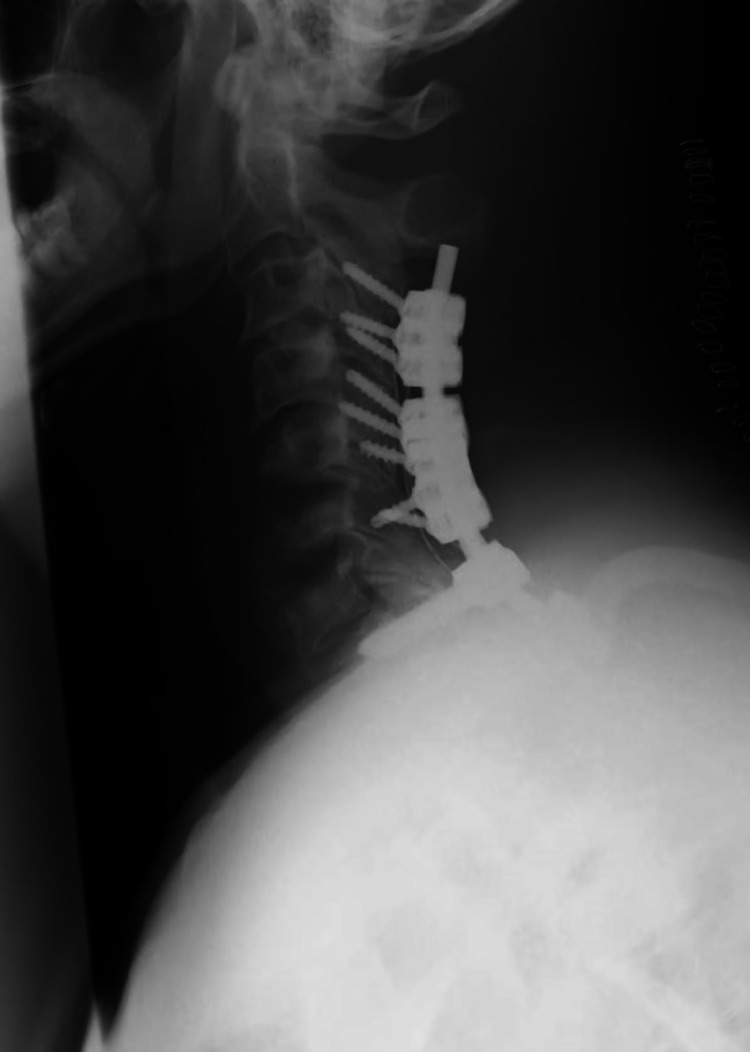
Cervical radiography after the second surgery Lateral view of the cervical radiograph after posterior fixation. Lateral mass screws were placed bilaterally at C3, C4, and C5 and on the left side at C6. A transarticular screw was placed on the right side at C6 due to the small lateral mass. Pedicle screws were placed bilaterally at Th1 and Th2. The pedicle screws at C7 were skipped because of pedicle fragility due to the spread of infection.
